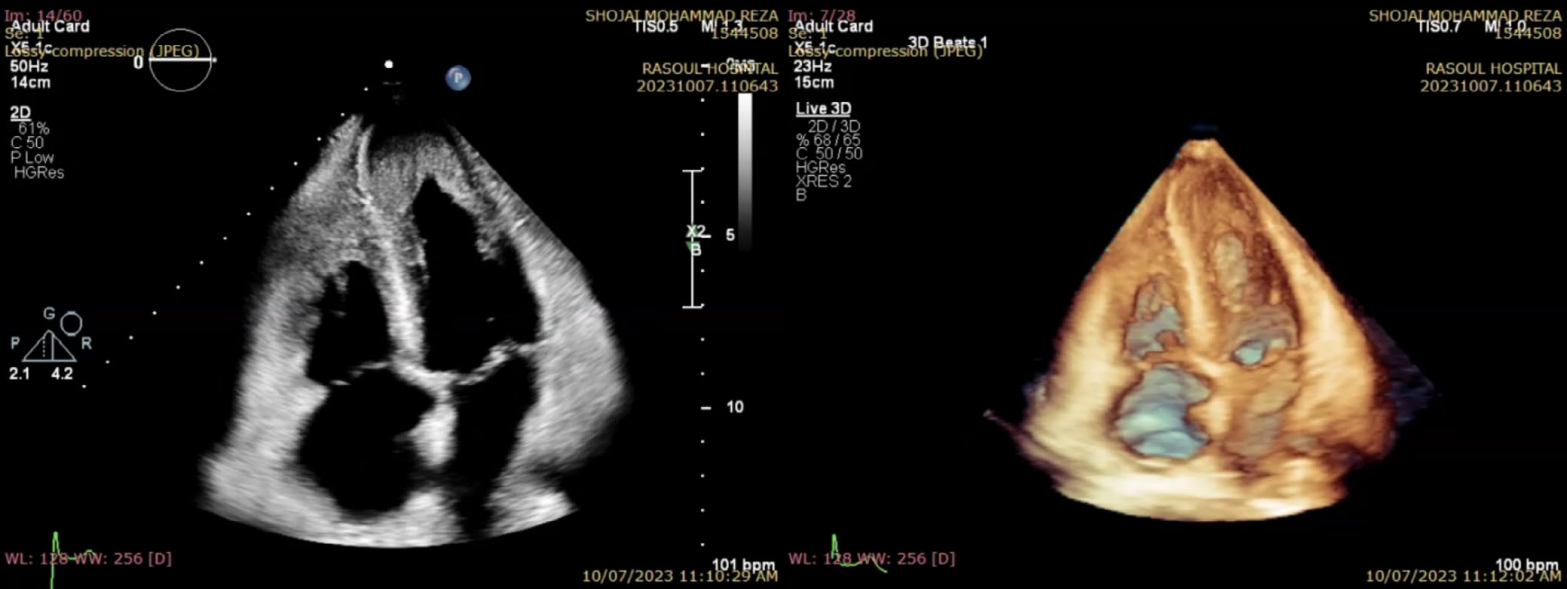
Apical 4‐chamber view of 2D‐TTE (left) and live 3D echocardiography showing obliteration of LV and RV cavities (right).
